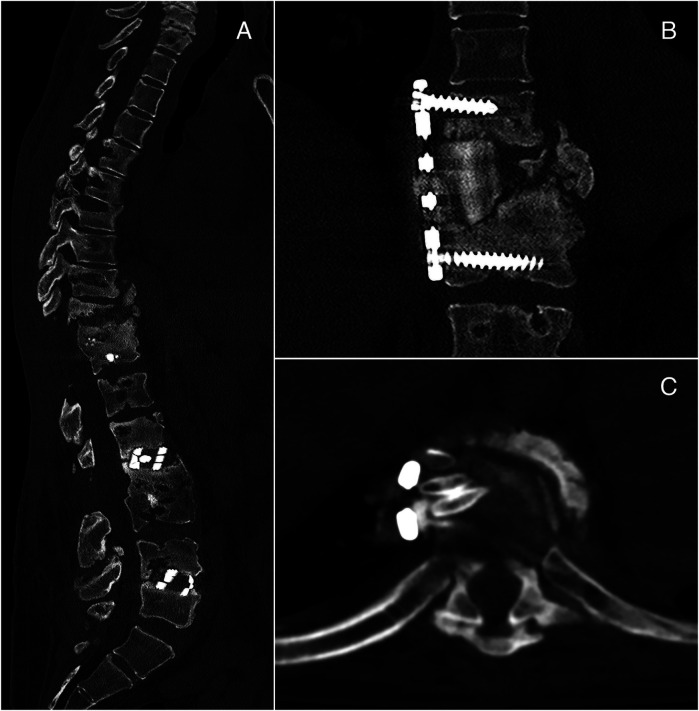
Left: thoracic compression and kyphosis at T10–T11 (A). Right: postoperative CT showing dorsal arthrodesis with plate and screws and rib autograft (B,C).
